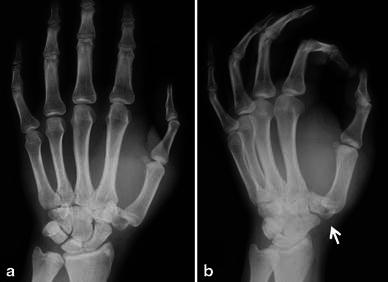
Anteroposterior (a) and oblique (b) radiographs. *White arrow* trapezium fracture
